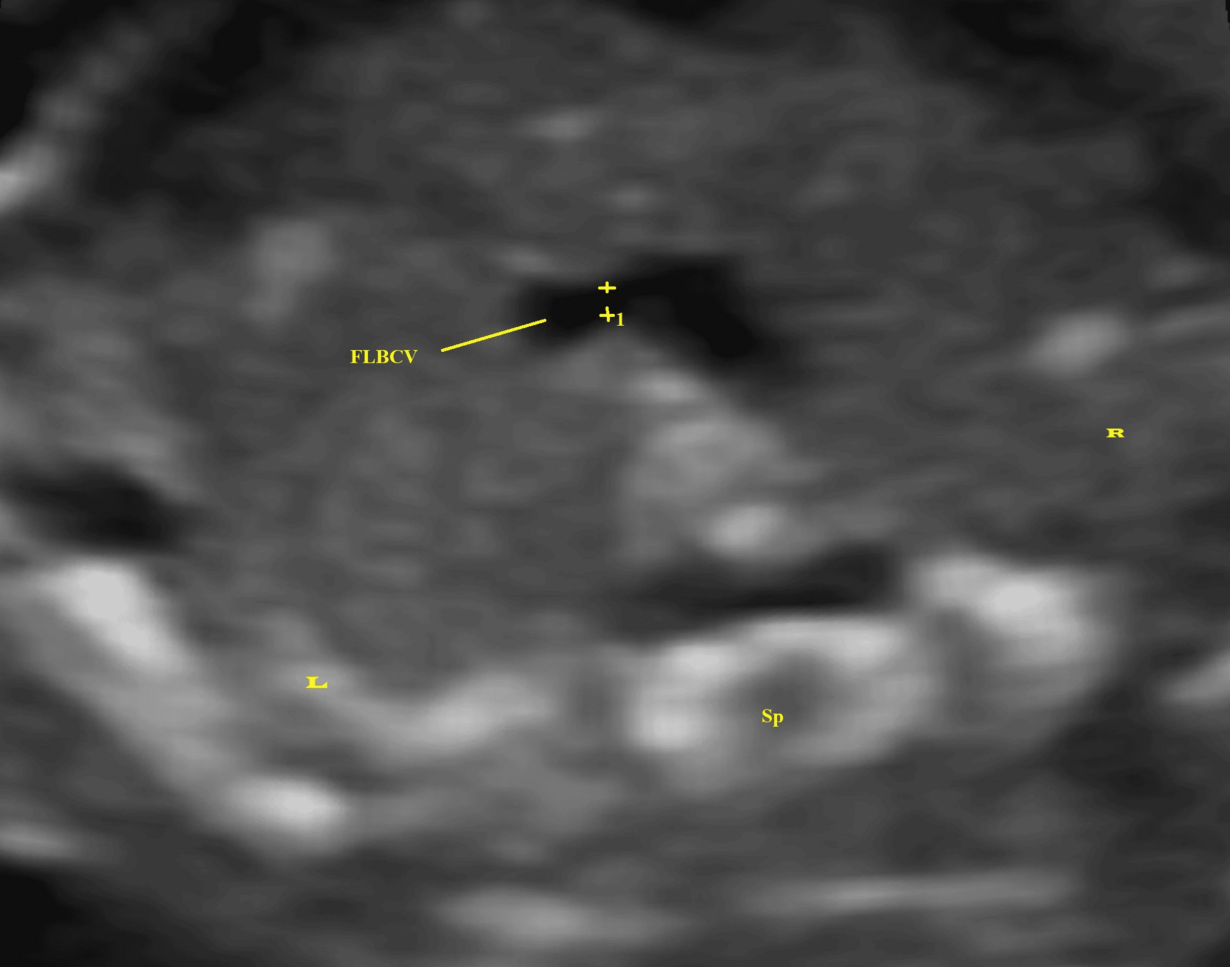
2D ultrasonographic image of the fetus showing the anteroposterior diameter of the FLBCV (1) FLBCV: fetal left brachiocephalic vein, Sp: spine, L: left, R: right, 2D: two dimensional
